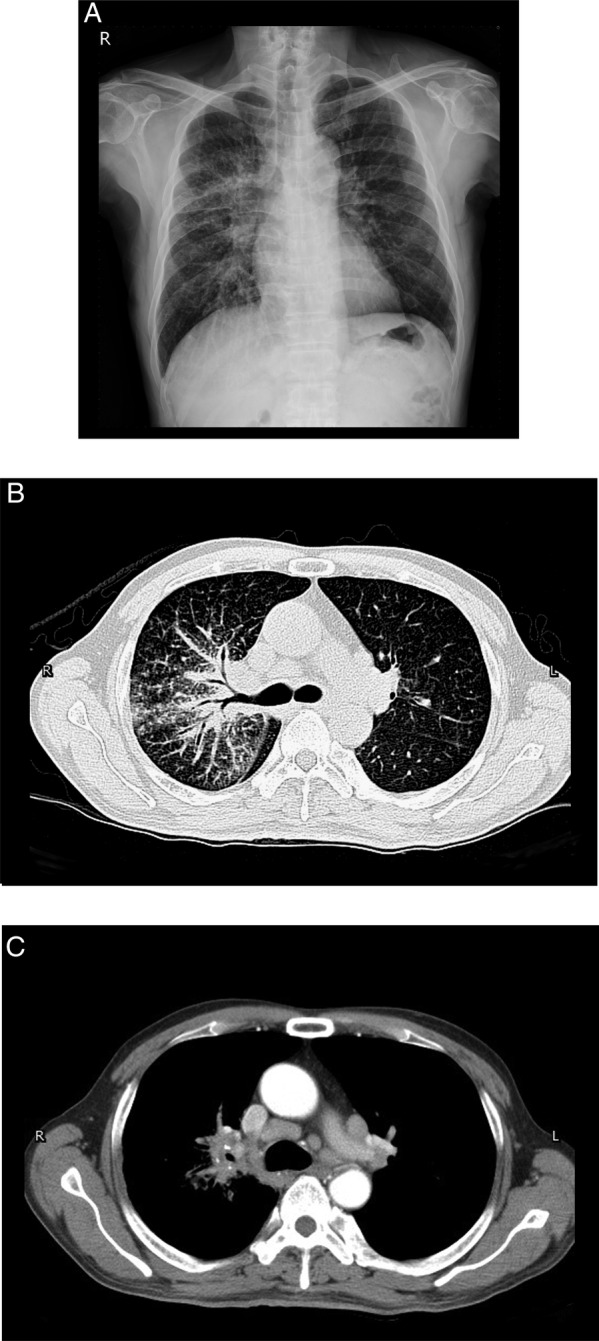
(A) Chest X‐ray showed increased interstitial marking right site, and patchy consolidations of the right lung. (B) Chest computed tomography (CT) showed unilateral small centrilobular nodules, and smooth peribronchovascular interstitial thickening, particularly in the right lung. (C) Contrast‐enhanced chest CT showed multiple enlarged mediastinal lymph nodes.
